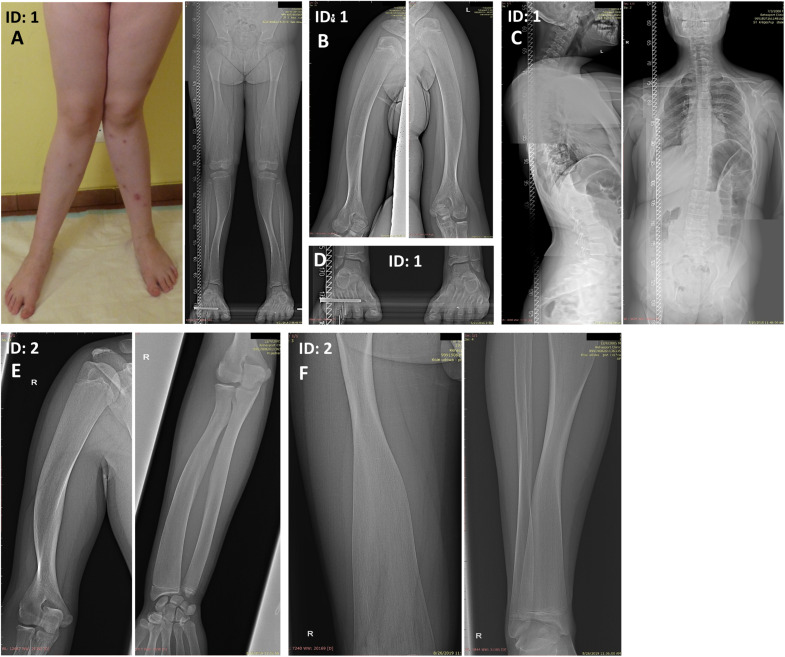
Skeletal malformations observed in the female proband (A–D) and her brother (E,F). (A) bilateral genua valga with a severe broadening of the knee joints. (A,F) Radiographs of the legs showing wide metaphyses and thin cortex of the femoral and tibial bones. (B,E) Radiographs of the upper extremities showing broadening of the proximal two-thirds of the humeri and the distal portion of the radial bone. The inward deviation of the distal one-third of the humeri was also noted (E). (C) Radiographs of the spine showing thoracolumbar scoliosis. (D) Radiograph of the feet showing broadened metatarsals. ID: 1, proband, ID: 2, affected sibling.
